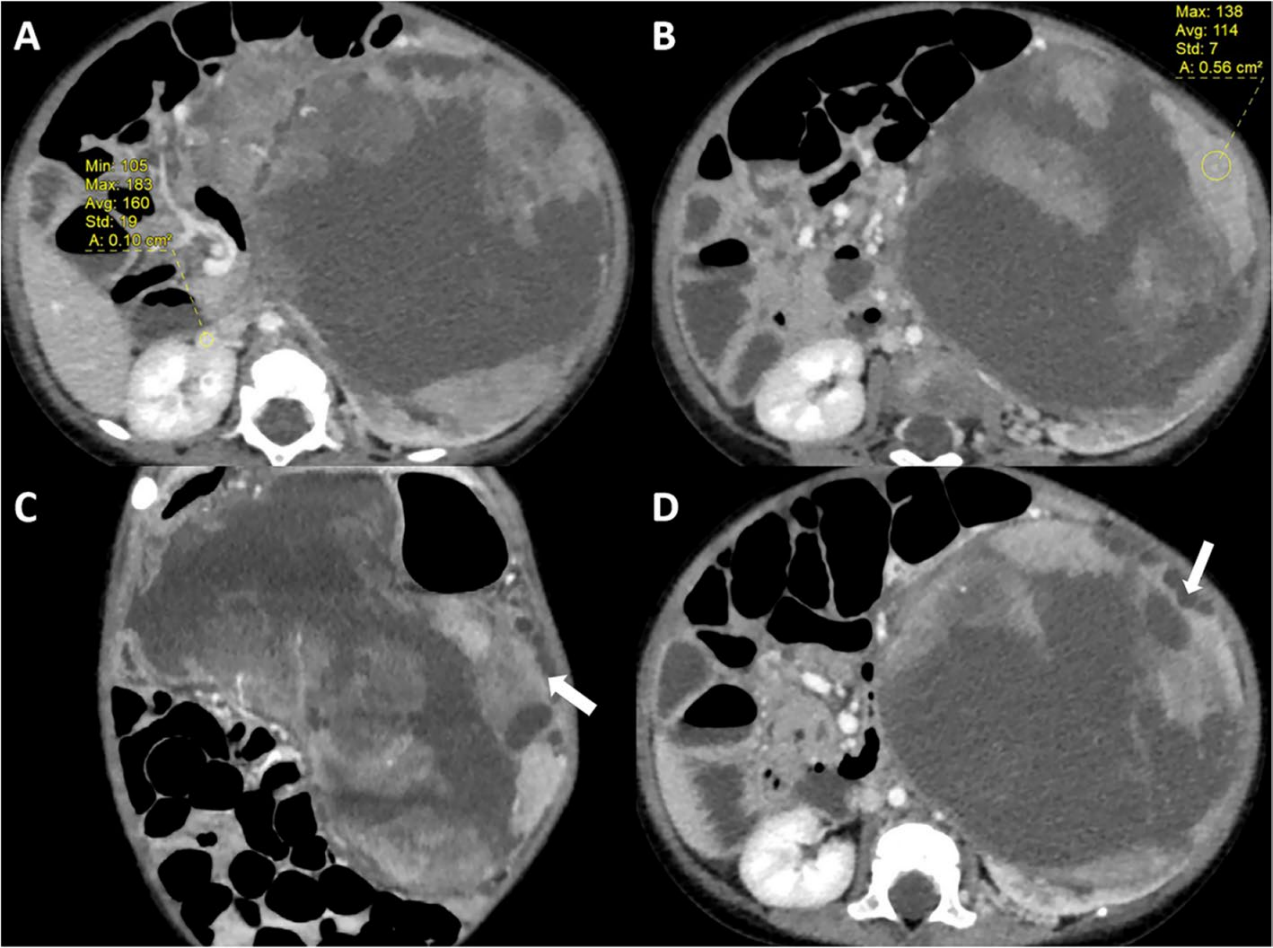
Examples of image analysis. Figure A shows the placement of a circular region of interest on the renal vein contralateral to the mass for measuring CT values; Figure B shows the placement of a circular region of interest on the most obviously enhanced part of the tumor parenchyma for measuring CT values; Figures C and D show the presence of dilated peritumoral cysts (white arrows)
